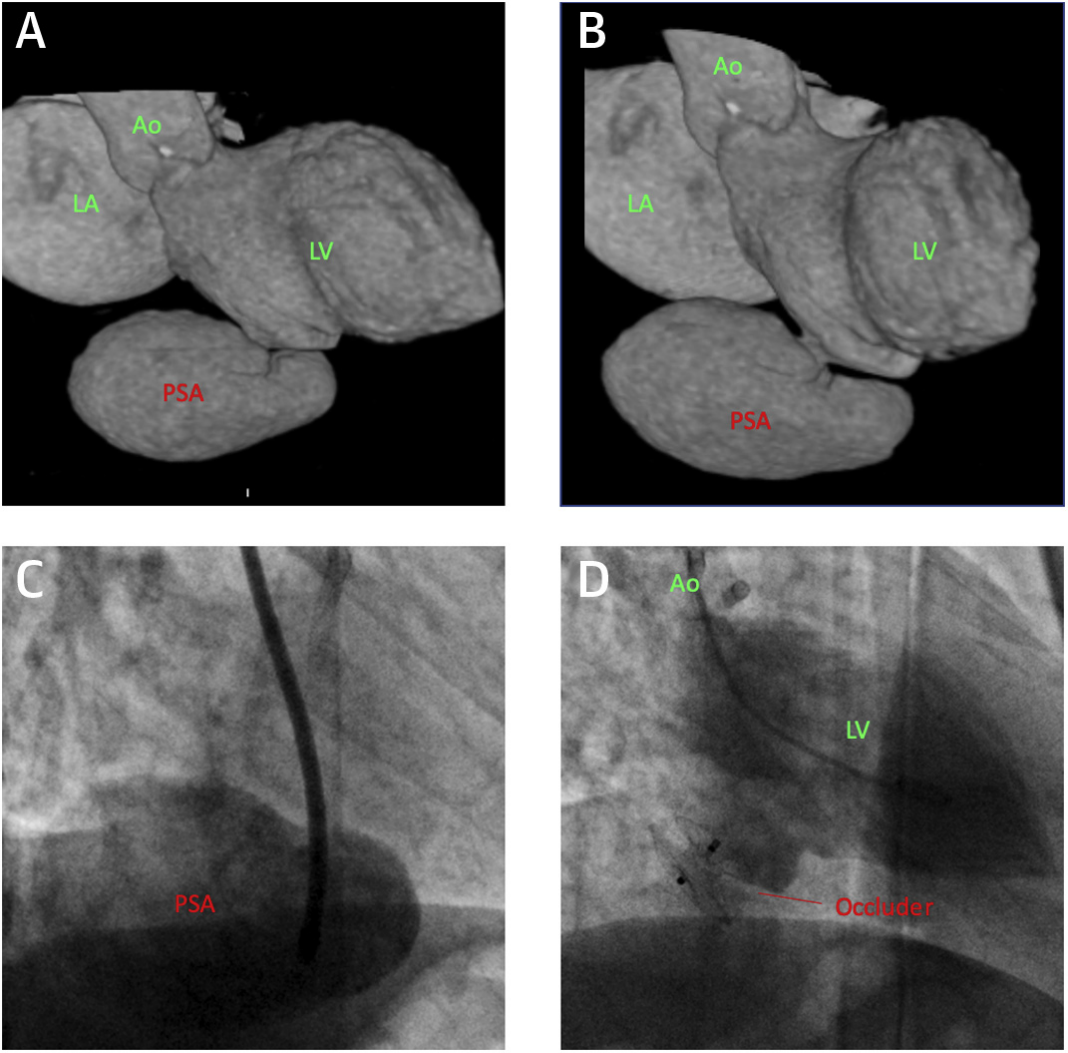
Left Ventricular Inferobasal Pseudoaneurysm (A and B) Volume-rendered computed tomography images of the left side of the heart showing the inferobasal pseudoaneurysm (PSA) with a narrow neck. (C) The anatomy is confirmed on angiography with contrast injection into the sac. (D) Successful occlusion of the pseudoaneurysm. Ao = aorta; LA = left atrium; LV = left ventricle.
